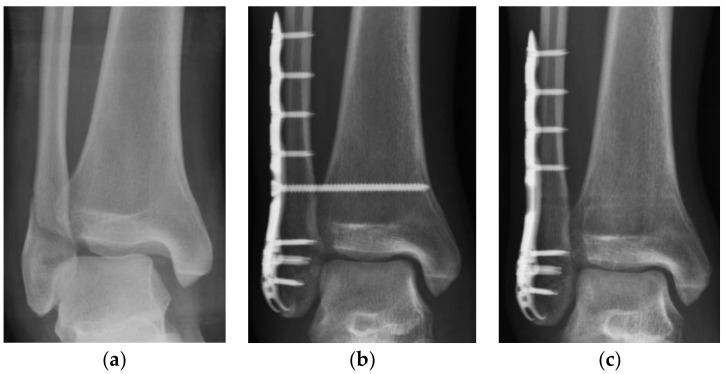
Demonstration of serial images evaluation: (a) Patients who met the inclusion criteria were collected; (b) pre-SR anteroposterior radiographs were obtained; (c) last-FU anteroposterior radiographs were obtained. CS: tibiofibular clear space; last-FU: last follow-up; OL: tibiofibular overlap; pre-SR: presyndesmotic screw removal.
